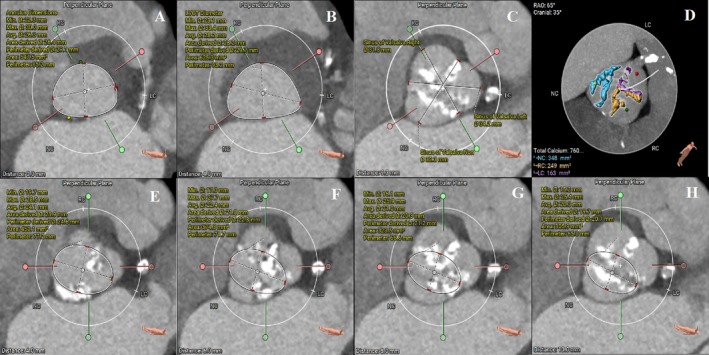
Preoperative computed tomography. (A–D) Tricuspid aortic valve with a 27.1 mm aortic annulus diameter, calcification masses with a total calcium score of 760 mm3. (E–H) Images 4, 6, 8, 10 mm above the annulus, respectively.
